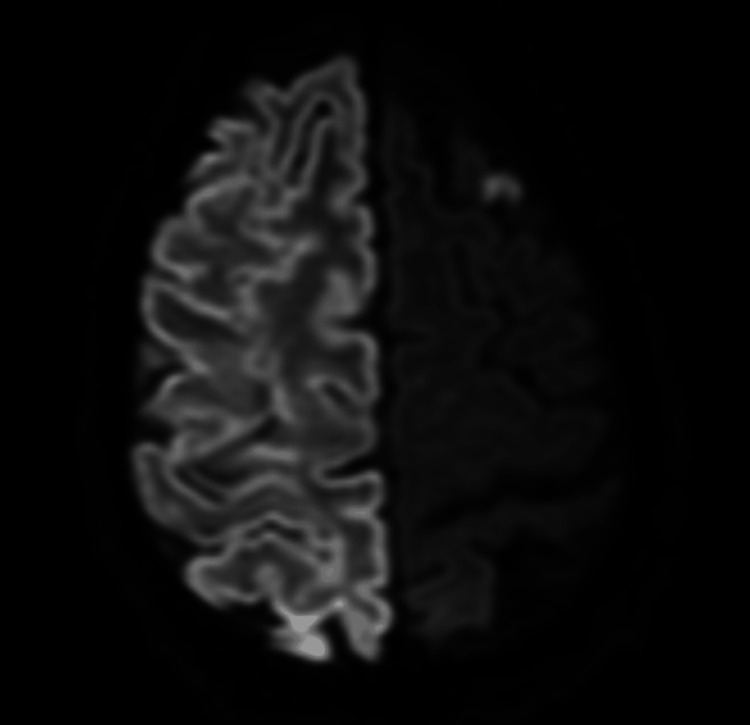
Brain MRI without contrast with diffusion-weighted imaging displaying lack of activity in the right hemisphere post-stroke MRI: magnetic resonance imaging
